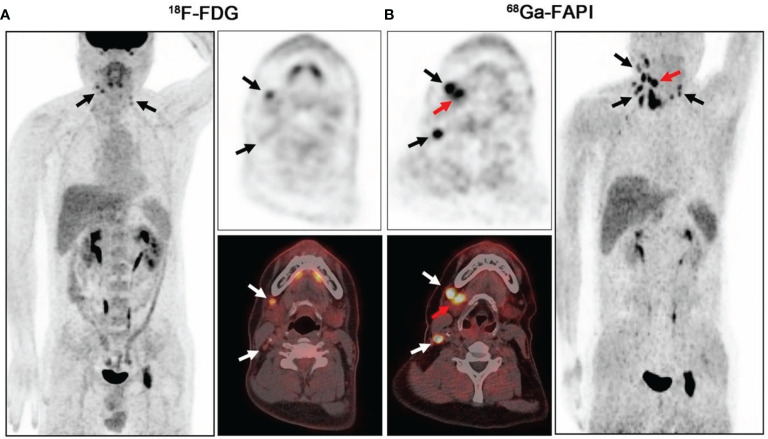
PET/CT scans of ^18^F-FDG (A) and ^68^Ga-FAPI (B) in a 41-year-old patient with lymph node metastasis from salivary gland ductal carcinoma. ^18^F-FDG-PET/CT was negative for the primary tumor. On ^68^Ga-FAPI-PET/CT, the right submandibular gland shows strong uptake (B, red arrow; SUVmax = 15.80), whereas the left submandibular gland has low background uptake (SUVmax = 6.87) (black and white arrows indicate lymph node metastases) (44).
